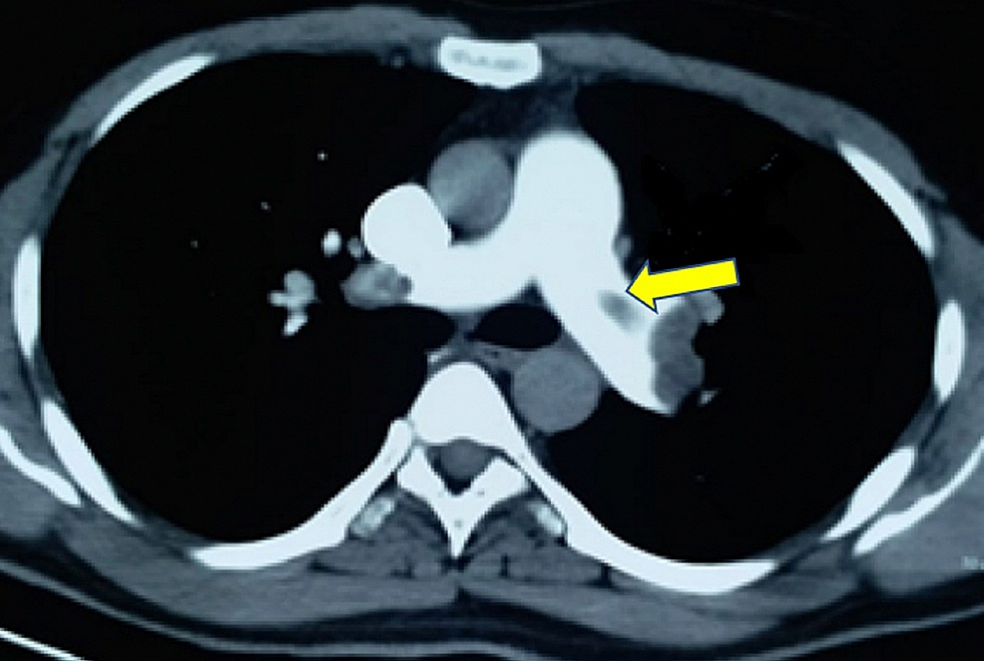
CT pulmonary angiogram showing thrombus in pulmonary artery
